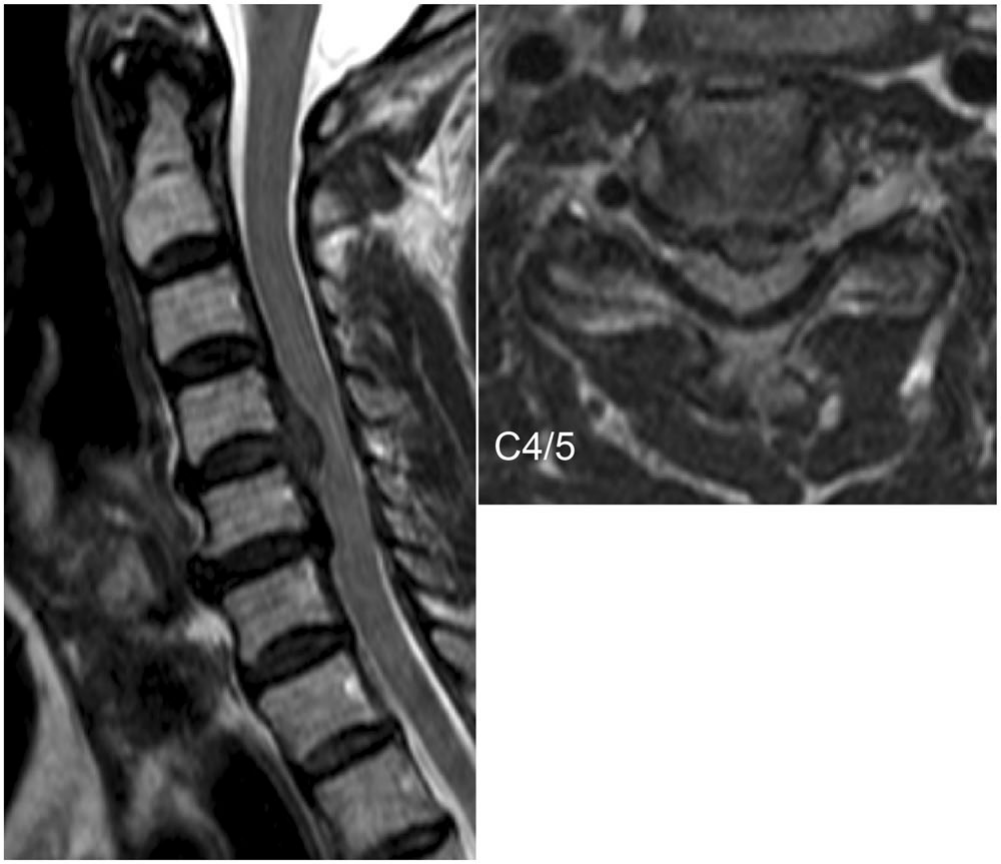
Preoperative MR image of a patient with cervical spondylotic myelopathy showing spinal stenosis. The patient was a 67-year-old male with C4/5 disc herniation and a small indentation of the C5/6 disc. The patient exhibited clumsiness of bilateral hands and gait disturbance.
